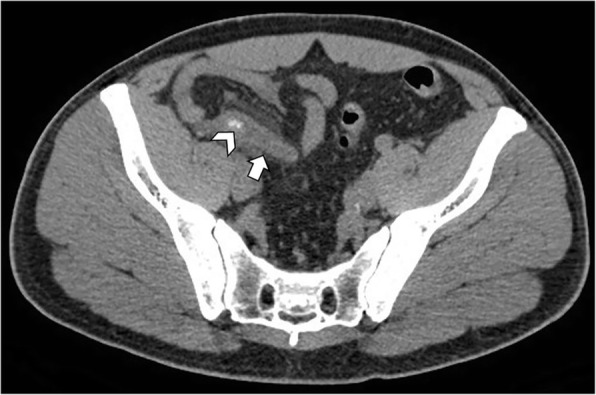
Acute appendicitis in a 43-year-old man. Axial non-contrast CT image shows an inflamed vermiform appendix (arrow), with thickened wall and nearby fat stranding. Appendicoliths are identified within the appendix (arrowhead)
